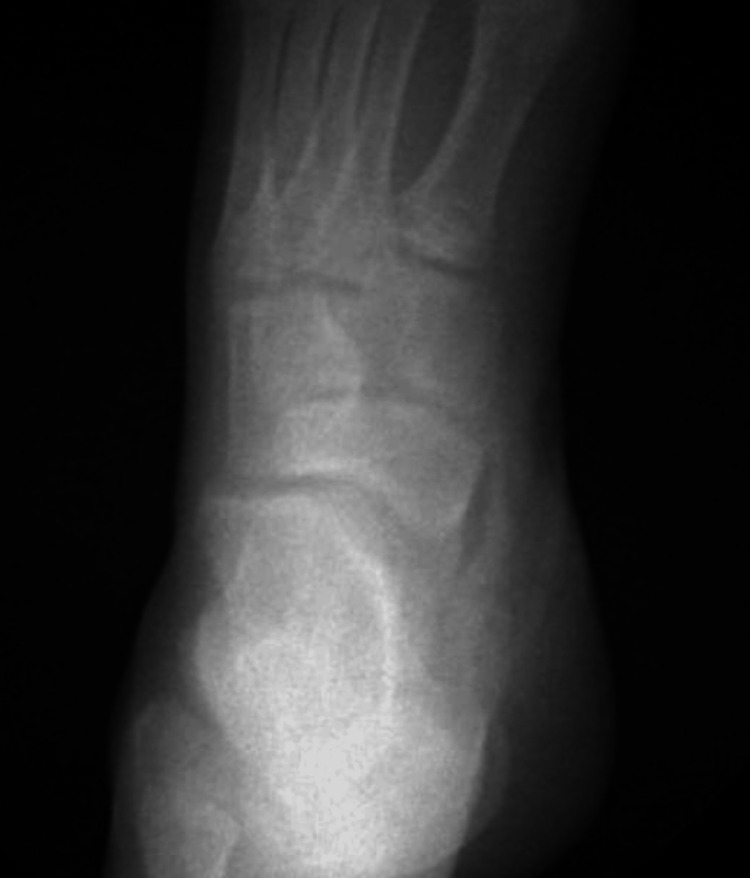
Intraoperative foot and ankle radiograph Intraoperative anterior-posterior (AP) view X-ray of the left foot following excision of the ossicles, demonstrating complete excision of the ossicles and no new osseous abnormalities.
